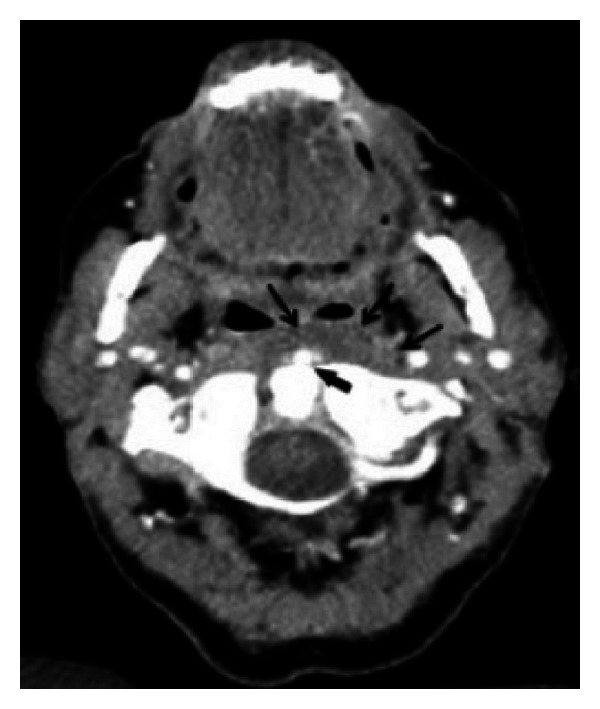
On the CT scan of the neck note the presence of an ill-defined calcification (thick black arrow) located in the left longus colli muscle at the level of C1. Note also the fat infiltration of the retropharyngeal space (thick black arrow).
